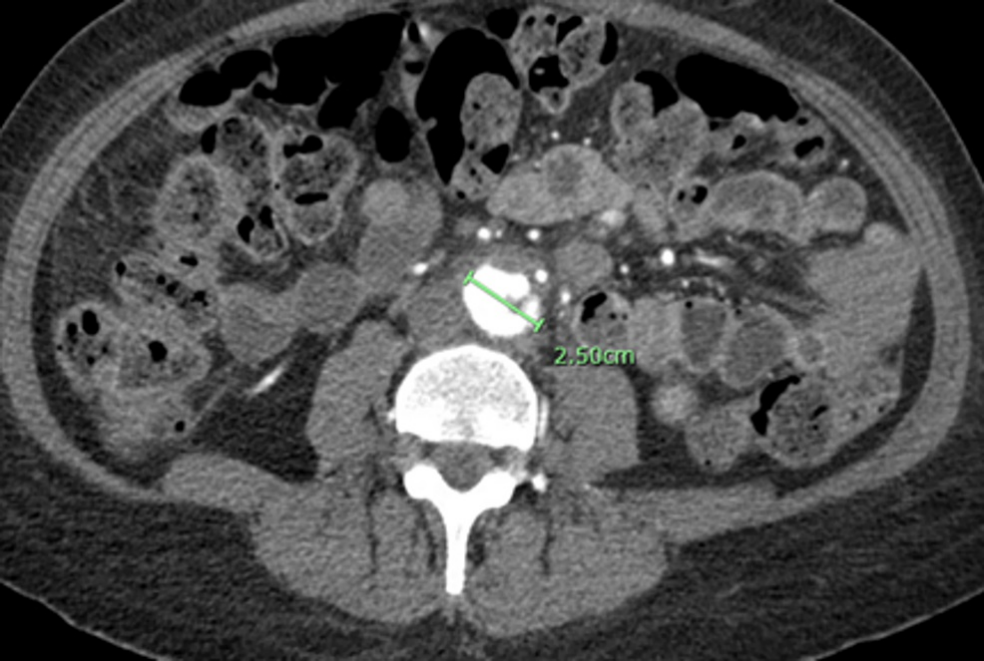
Cross-sectional CT abdomen with contrast showing 2.5 cm contained ruptured mycotic abdominal aneurysm
